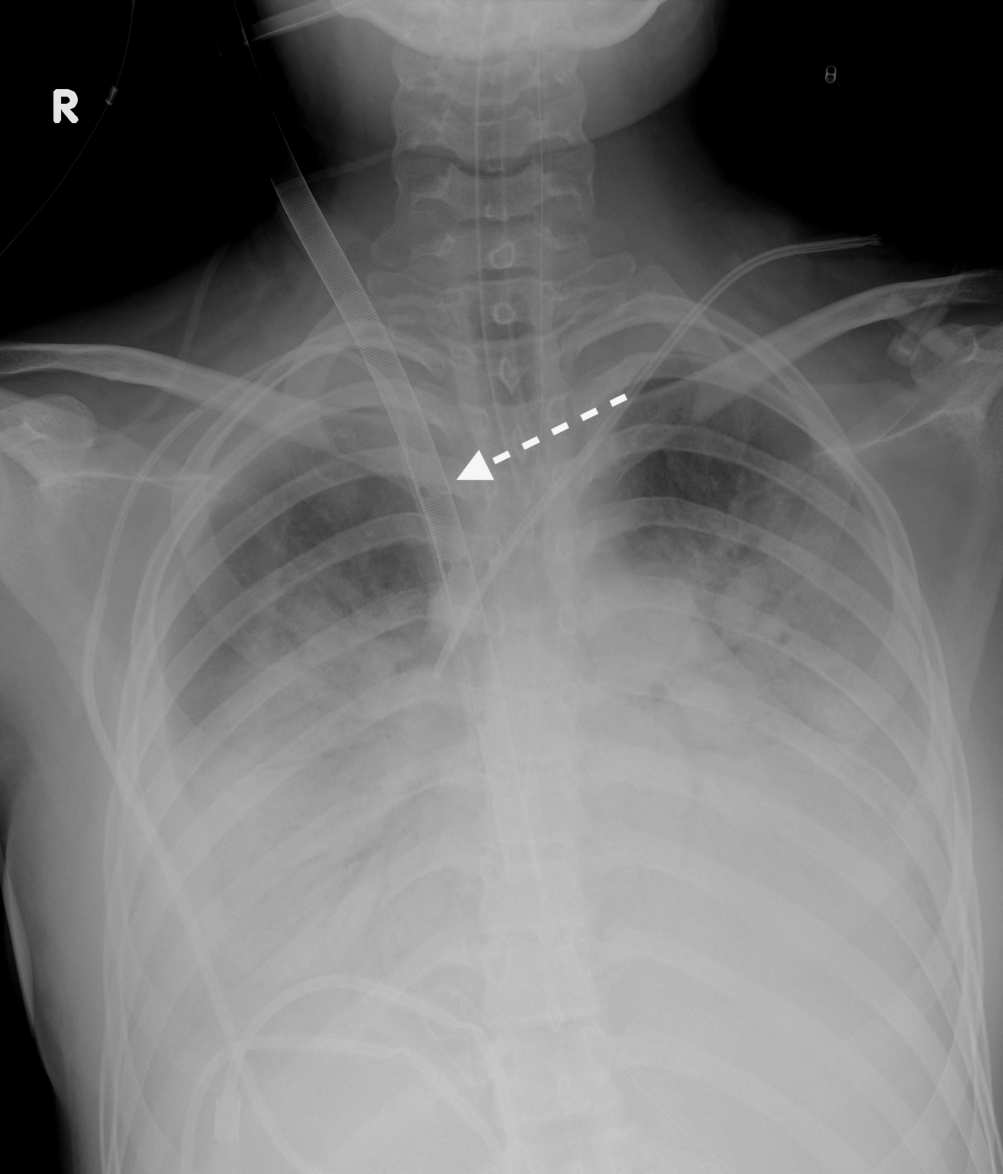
The X-ray after cannulas and central venous catheter (CVC) insertion The first side holes are positioned at the level of the clavicle.
